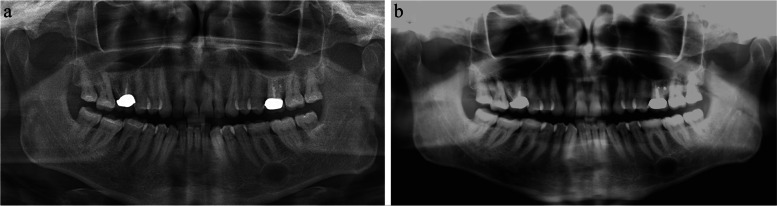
Panoramic radiographs demonstrating the intrabony lesion. a Panoramic radiograph 2017. b Panoramic radiograph 2011
